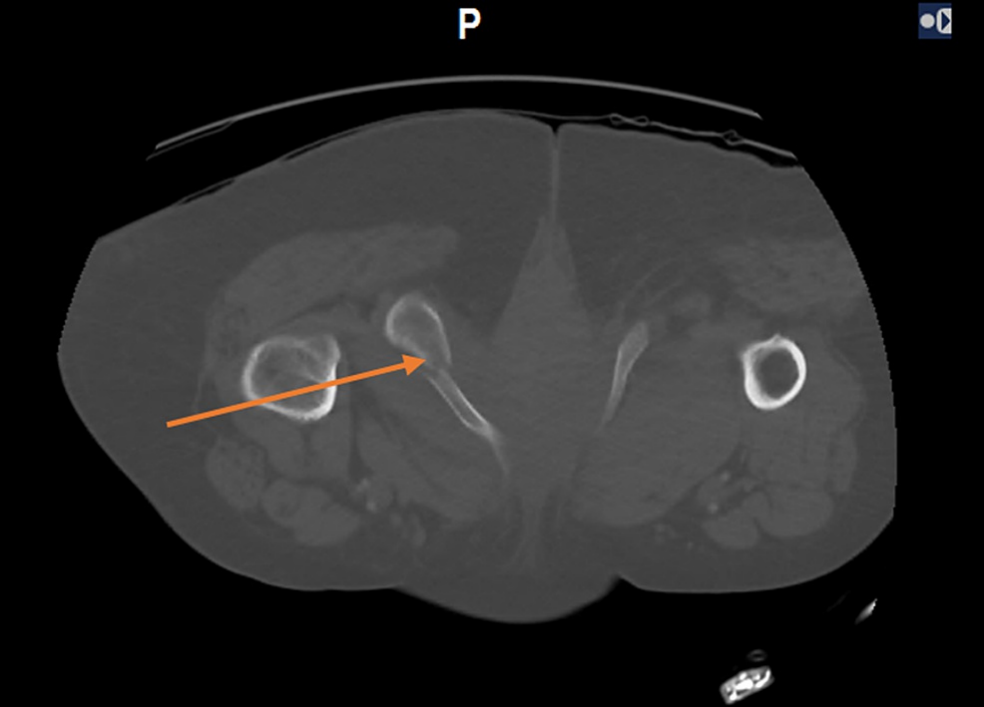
Initial pelvic CT showing an inferior pubic ramus fracture (arrow) of the pelvis
